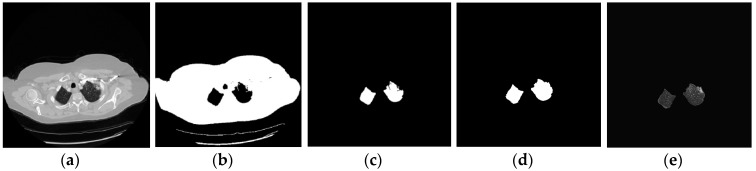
This is one successful example of lung extraction while the nodule is linked to the pleura (a) CT image showing all the structures; (b) Binary image after the threshold; (c) Lung selection for extraction with area loos; (d) Lung completed after the application hole-filling algorithm; (e) The lung extracted showing the internal structures.
